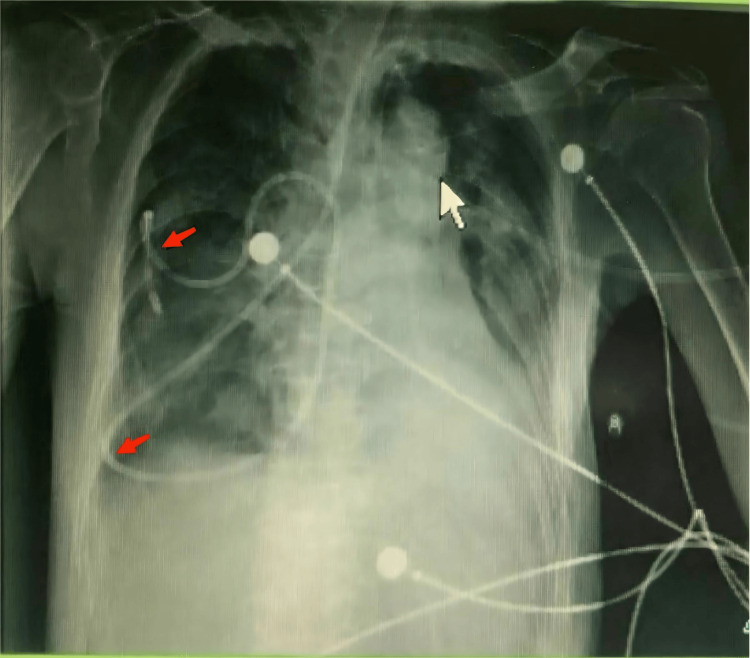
Bedside anteroposterior (AP) chest radiograph showing the naso-intestinal tube (red arrow) coiled in the right pleural cavity
